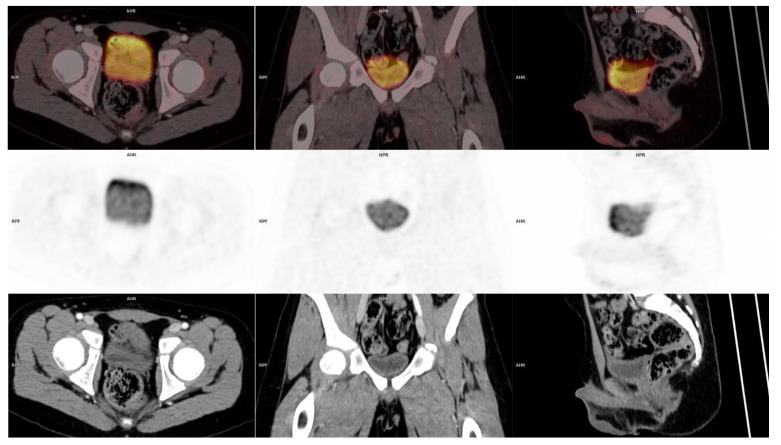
Follow-up: ^18^F-FDG-PET/CT fused images (top row) transaxial, coronal and sagittal view, PET images (middle row) and CT images (bottom row) where the lesion in the bladder wall is no longer visible.
